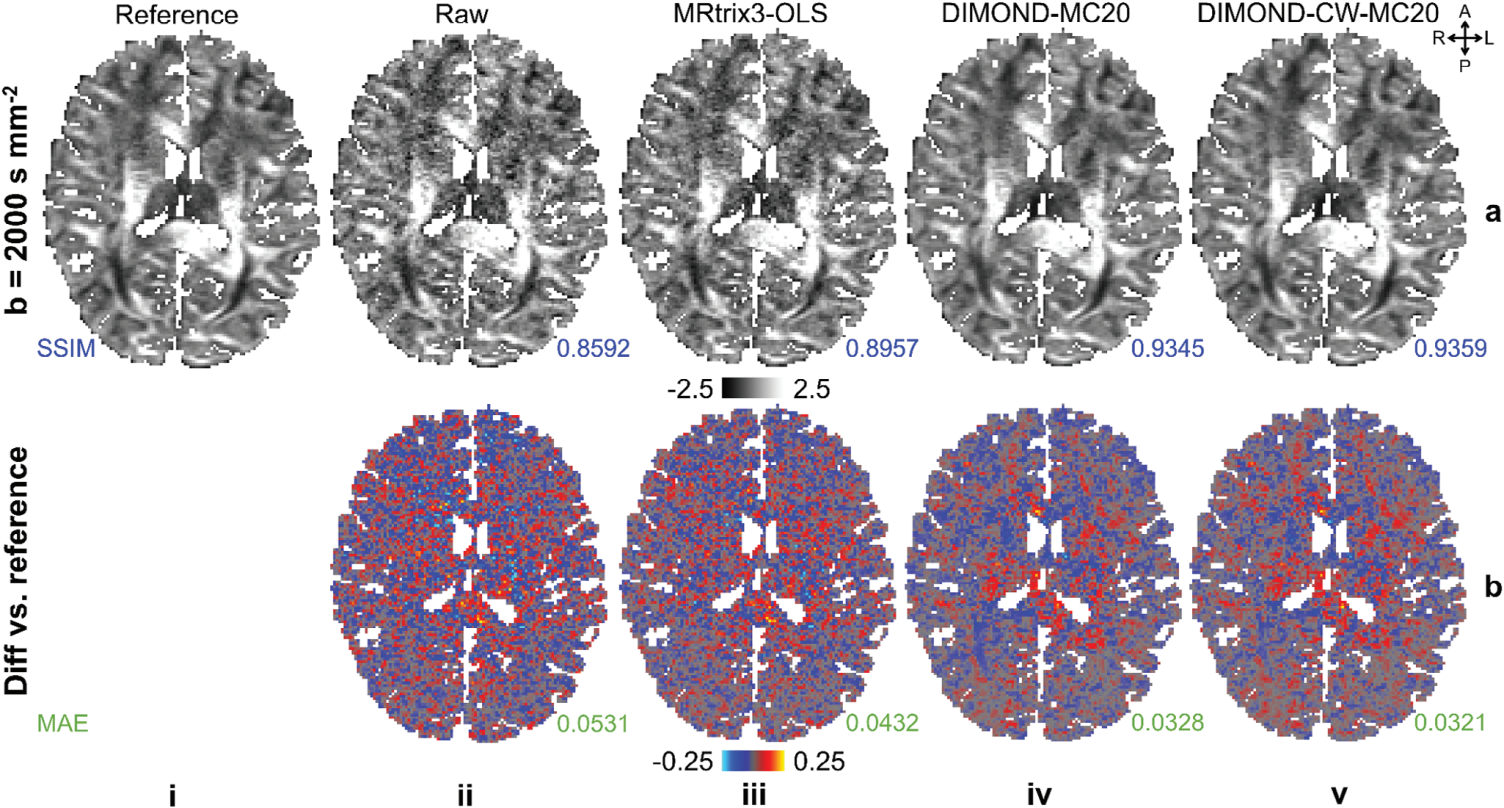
Images synthesized using kurtosis model. a) Exemplary axial image slices of diffusion‐weighted image (DWI) volumes (b = 2000 s mm^−2^ along [0.33, 0.87, 0.35]) from the reference data (a(i), synthesized using reference kurtoses via the kurtosis model), raw acquired data (a(ii)), and those synthesized from kurtoses generated using MRtrix3‐OLS (a(iii)), DIMOND‐MC20 (a(iv)), and DIMOND‐CW‐MC20 (a(v)) from sub‐sampled data of a representative HCP subject are shown. b) Difference maps between the reference and resultant DWIs are displayed, with structural similarity indices (SSIM, blue) and mean absolute errors (MAE, green) listed to quantify the difference.
